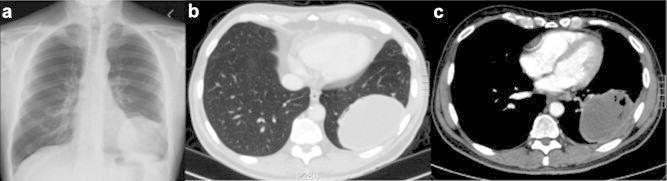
(a) CXR on presentation to our hospital (b) CT imaging -lung window (c) CT imaging -soft tissue window.
